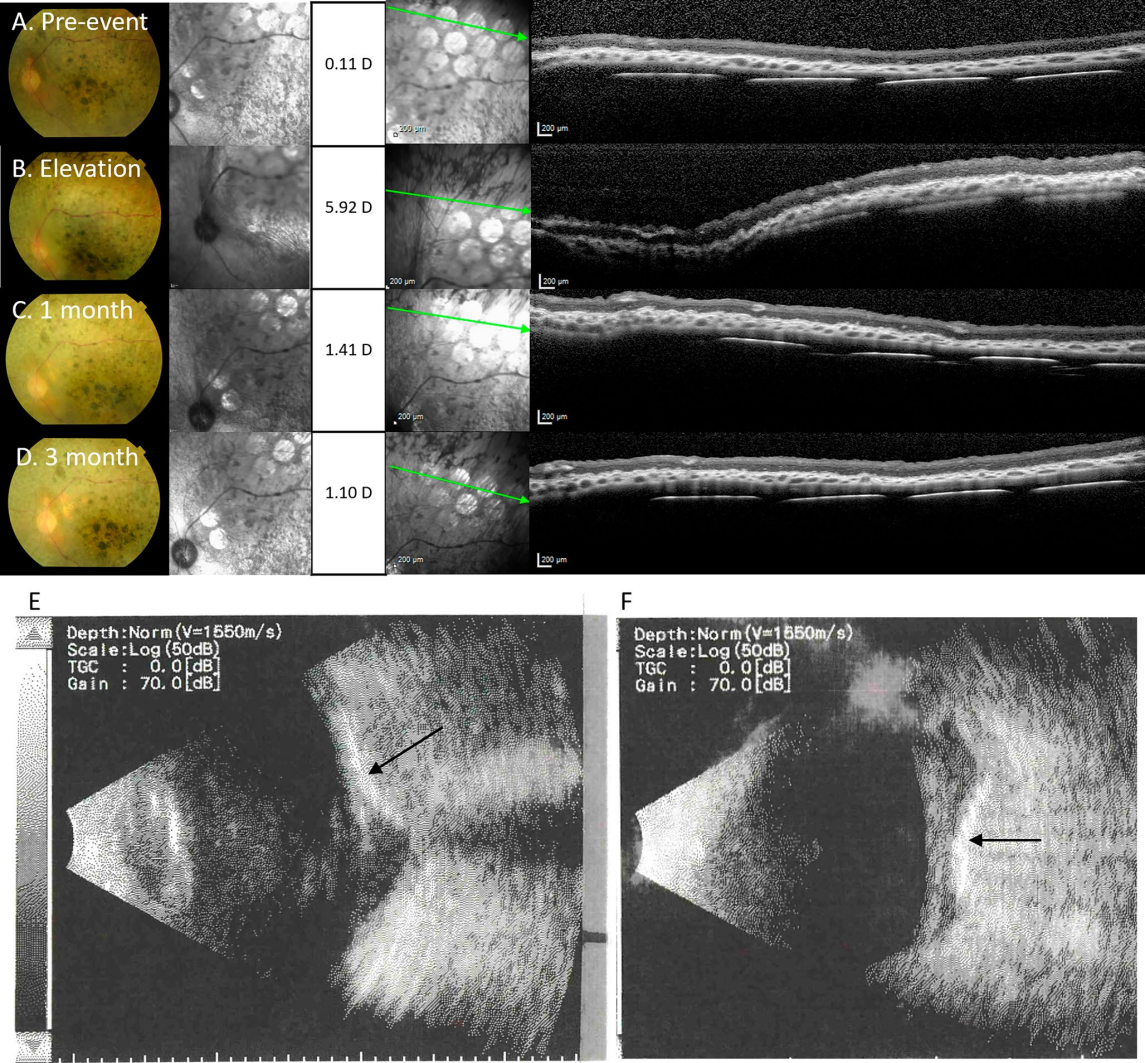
Choroidal effusion event in S1 noted at 2.5 months post‐implantation. (A–D) Colour fundus photos, near infrared images, the focus setting in Dioptres on the Heidelberg Spectralis and the OCT B‐scan image through the superior row of the electrode array, showing elevation of the array and overlying retina and the subsequent recovery over 3‐months ((A) at pre‐event, (B) retinal elevation during event, (C) 1‐month after event onset, (D) 3‐months after event onset). (E) B‐scan ultrasound positioned through optic nerve. (F) B‐scan ultrasound positioned temporal to optic nerve. Shadows posterior to the array are cast by the platinum electrodes. There was no sign of retinal, subretinal or suprachoroidal haemorrhage (no attenuation of ultrasound signal), hence the event was diagnosed as a choroidal effusion that resolved without treatment. Arrows indicate the position of electrode array within the suprachoroidal space.
